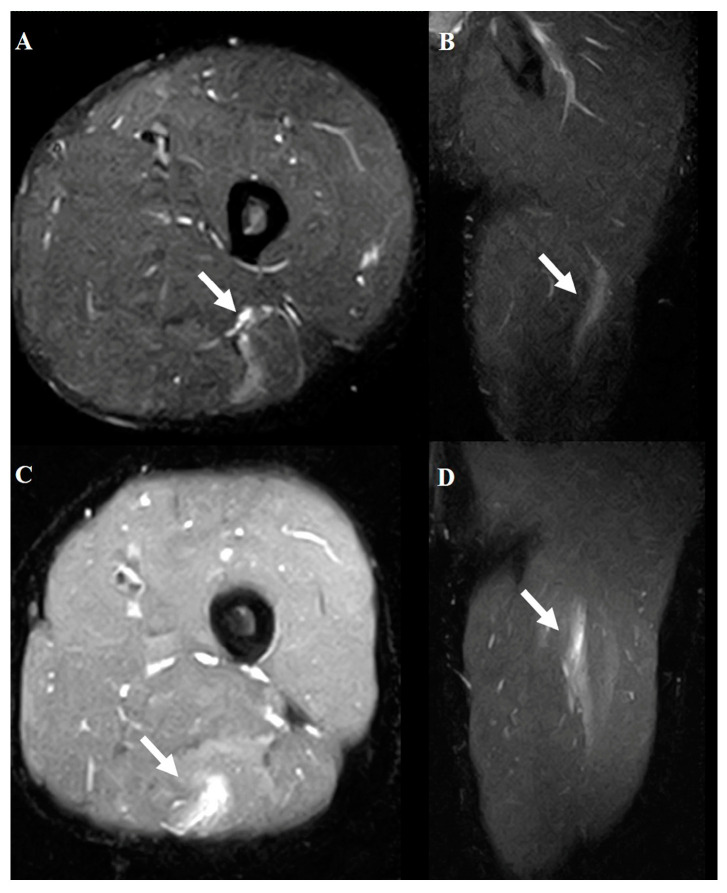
Typical MRI findings in acute hamstring strains. Axial (A) and coronal (B) views show a grade 1 injury of the biceps femoris muscle. Axial (C) and coronal (D) views show a grade 2 injury of the semitendinosus muscle. The white arrows indicate the injured areas.
